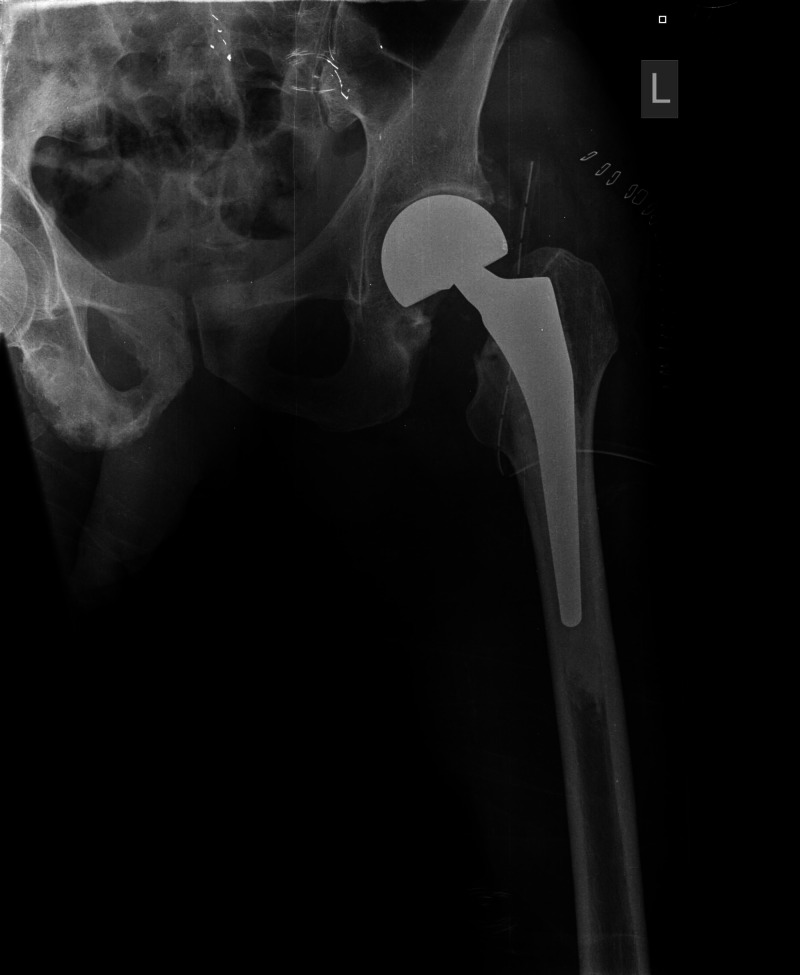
Postoperative anteroposterior radiograph of the left hip showing the left hip cemented bipolar hemiarthroplasty
